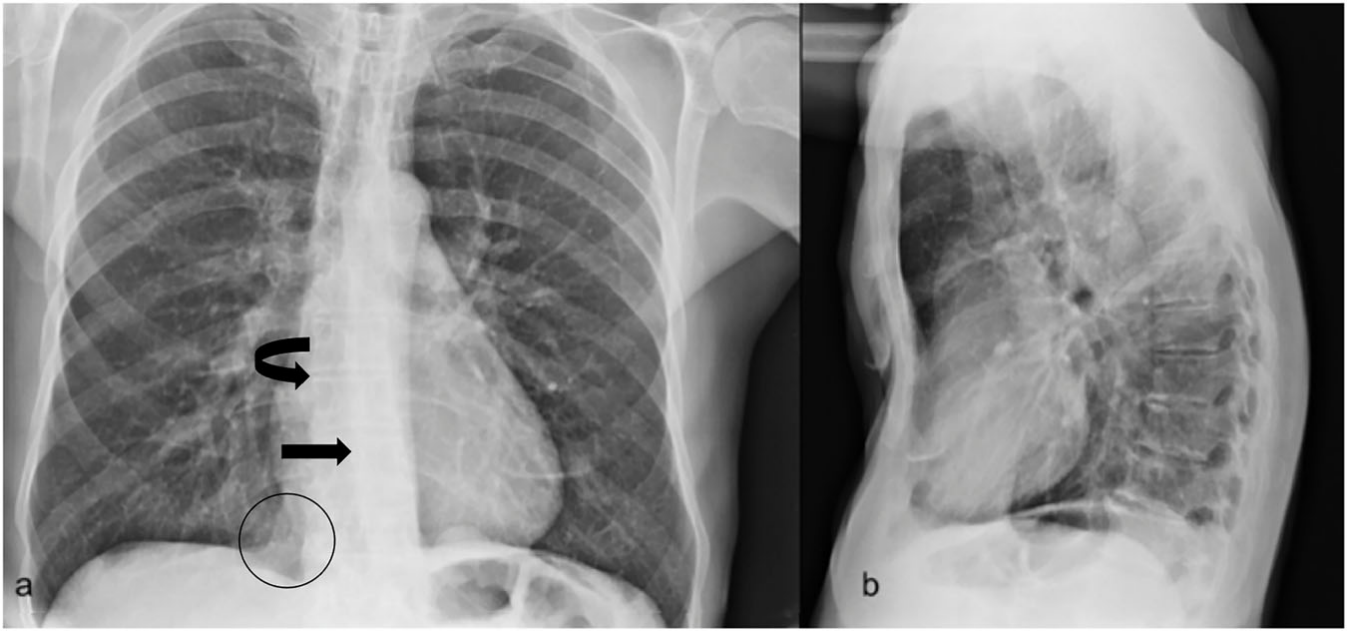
Chest X-ray was performed in orthostatism in two standard projections. This is a case of a 61-year-old male patient with a history of cardiac arrythmia. The posteroanterior projection (a) shows increased inferiorly-medial right hypodiaphany, related to increased visibility of the parasternal soft tissues located in the anterior chest wall (black circle), blurring of the right cardiac margin (curved black arrow) and axial rotation of heart with dislocation to the left side in the corresponding area of descending aorta (black arrow); on lateral view, the degree of depression could be easily assessed (b)
